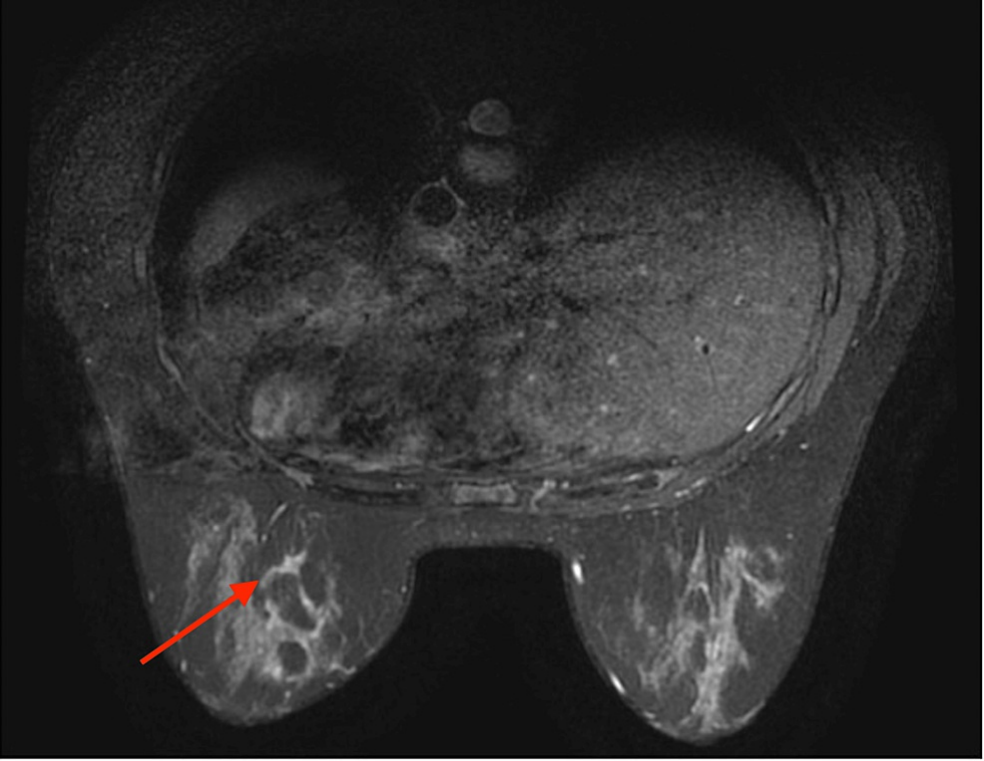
Follow-up breast magnetic resonance imaging (T2-weighted imaging). Regional non-mass enhancement was shrinking but still present as indicated by the arrow. The lesion was classified as breast imaging reporting & data system (BI-RADS) category 4A.
